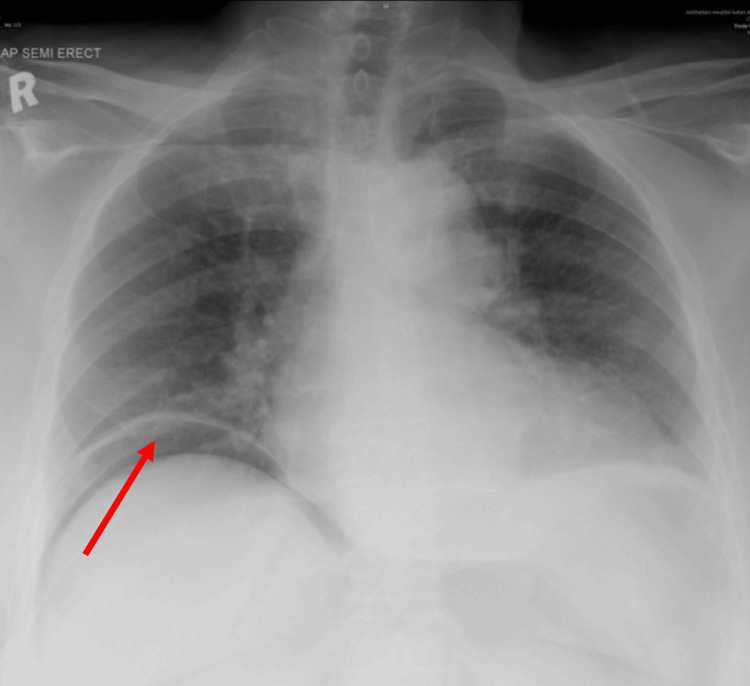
Chest radiograph demonstrating air under the right hemidiaphragm (arrow), consistent with pneumoperitoneum.
